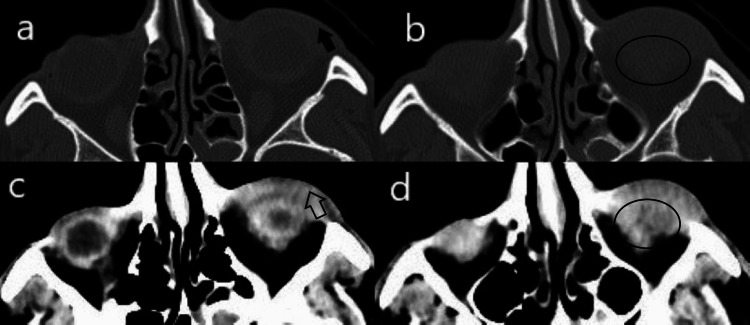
CT scan showing soft tissue mass lesion in left lower eyelid (arrow) with intraorbital extension (circle) in (a, b) thin bone and (c, d) thin plain axial section
